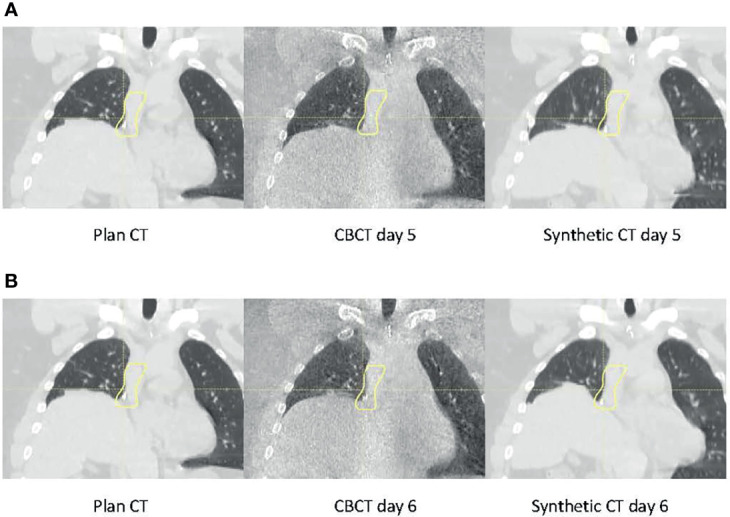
Planning CT (left), daily cone-beam CT (CBCT (middle), and synthetic CT (right) for the same patient on (A) day 5 and (B) day 6.
